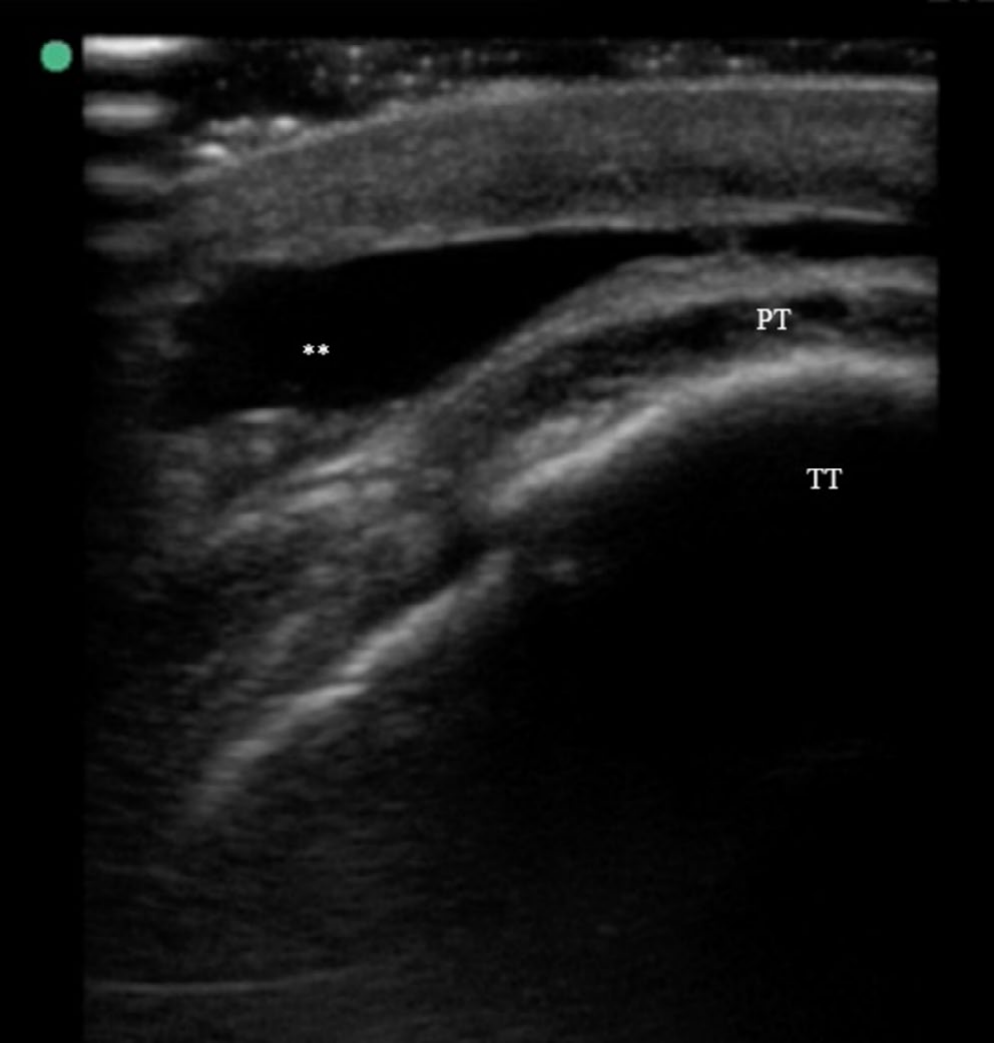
Point‐of‐care ultrasound short axis view over the tibial tuberosity demonstrating fluid collection. PT, distal patellar tendon; TT, tibial tuberosity. : Subcutaneous fluid collection consistent with a Morel‐Lavallée lesion.
